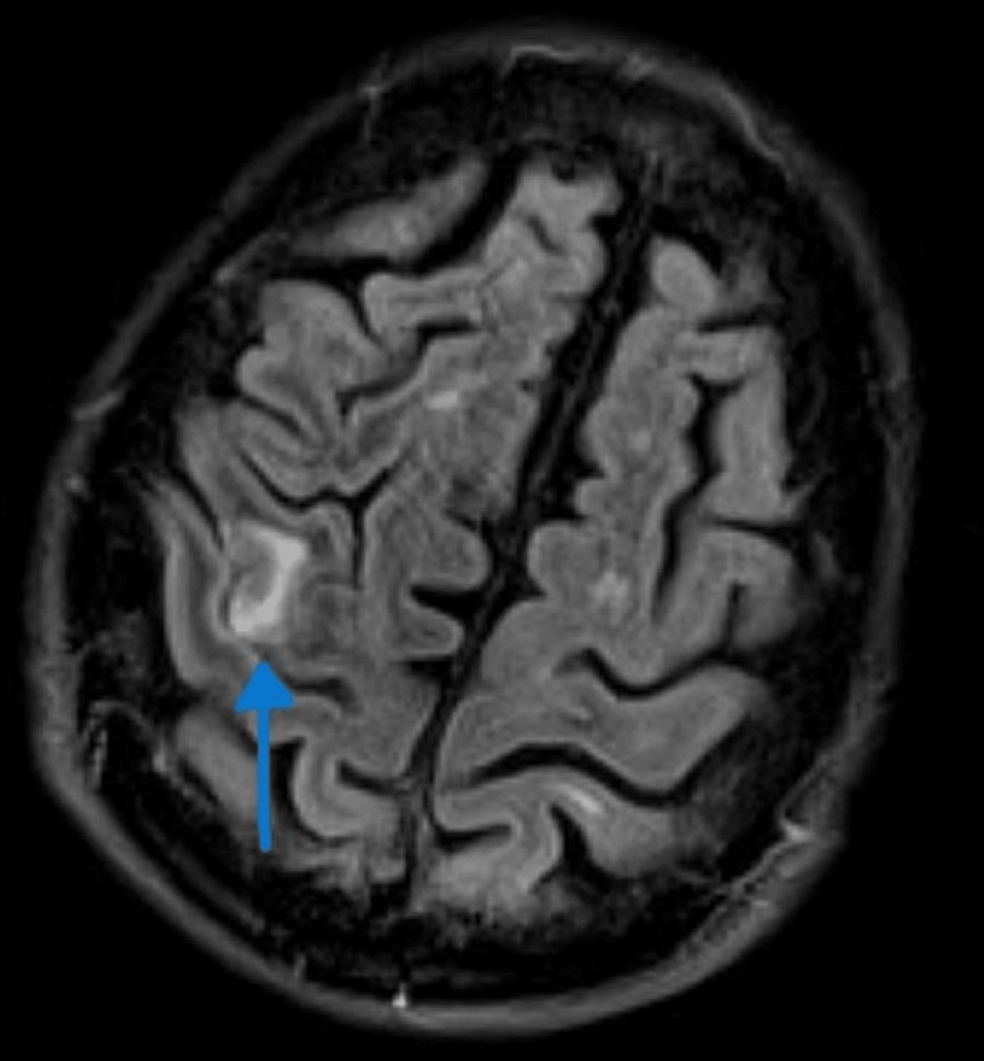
Brain MRI Axial T2 FLAIR weighted images showed an ischemic lesion in the right frontoparietal region. FLAIR: fluid-attenuated inversion recovery
